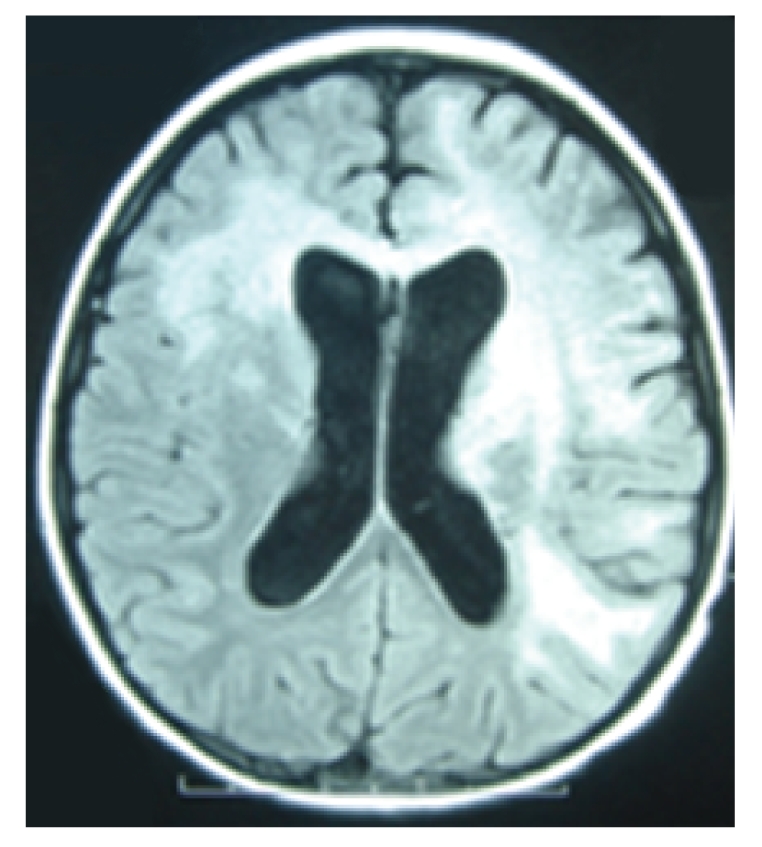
MRI on day 38 of onset of symptoms and 2 weeks post-HAART initiation.
